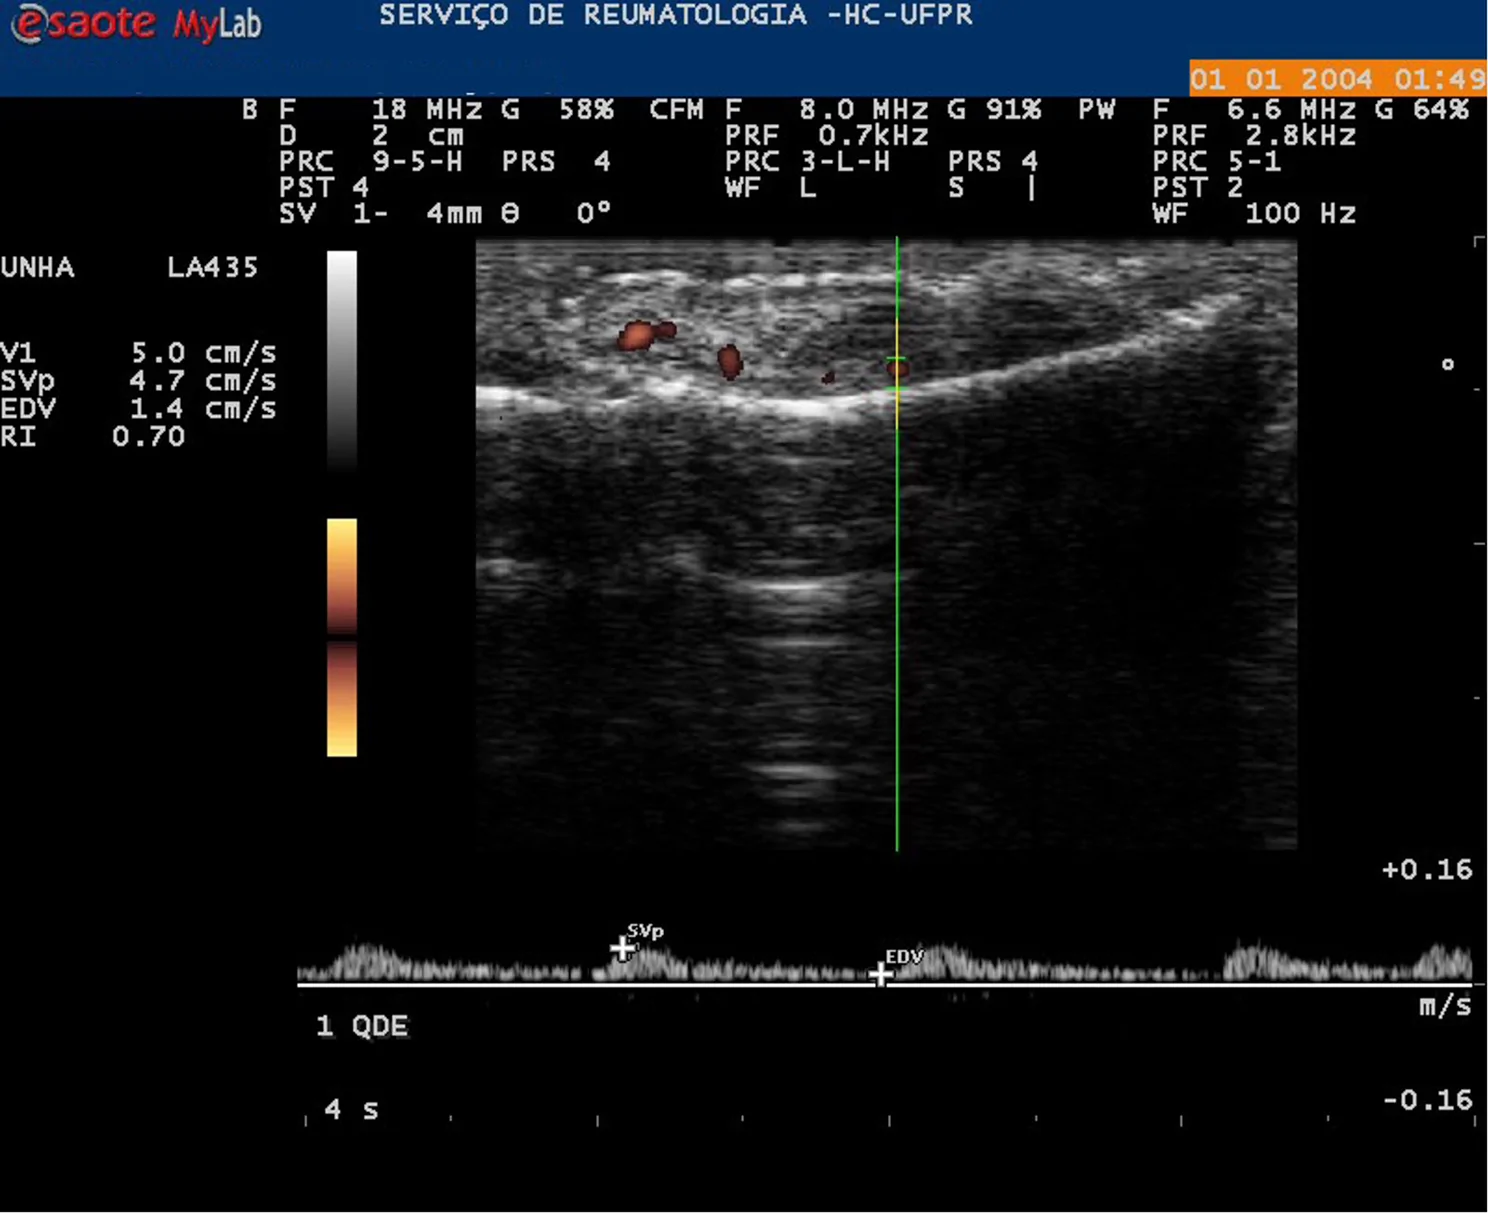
Power doppler imaging and calculation of the RI (resistivity index) of the nail bed. *Notes* Power doppler of the nail bed grade 1. Vessel resistivity index 0.70
